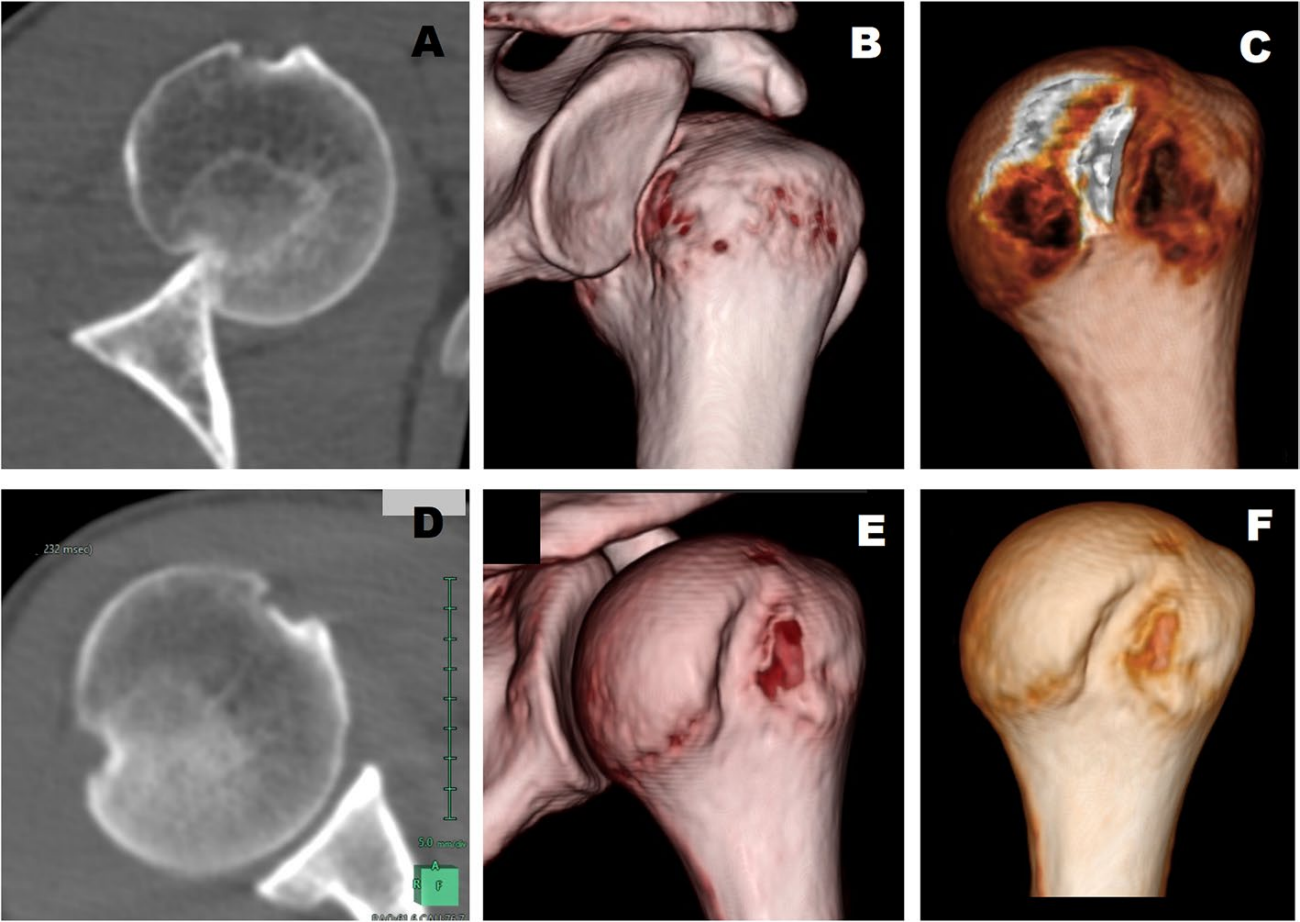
Prereduction and postreduction CT scans of one of the cases (Case 8 in Fig. 2). The prereduction images (A, B and C) show the dislocated shoulder and the small Hill- Sachs lesion. Postreduction CT images (D, E and F) show a moderate deepening of the defect
